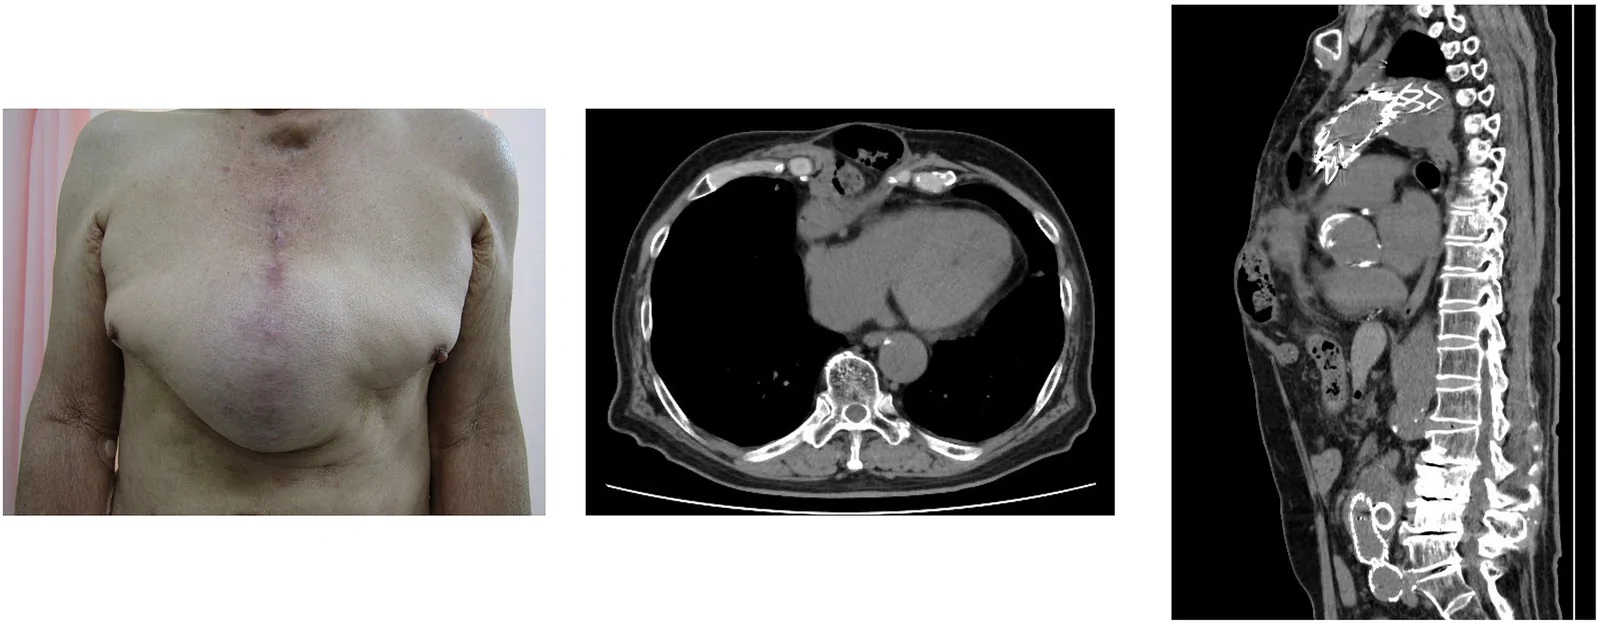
Preoperative findings of iatrogenic diaphragmatic hernia following pedicled omentoplasty. Left: Clinical photograph demonstrating prominent bulging of the anterior chest wall. Center: Axial CT image showing herniation of the transverse colon into the anterior mediastinum. Right: Sagittal CT image confirming the herniation through the diaphragmatic defect.
